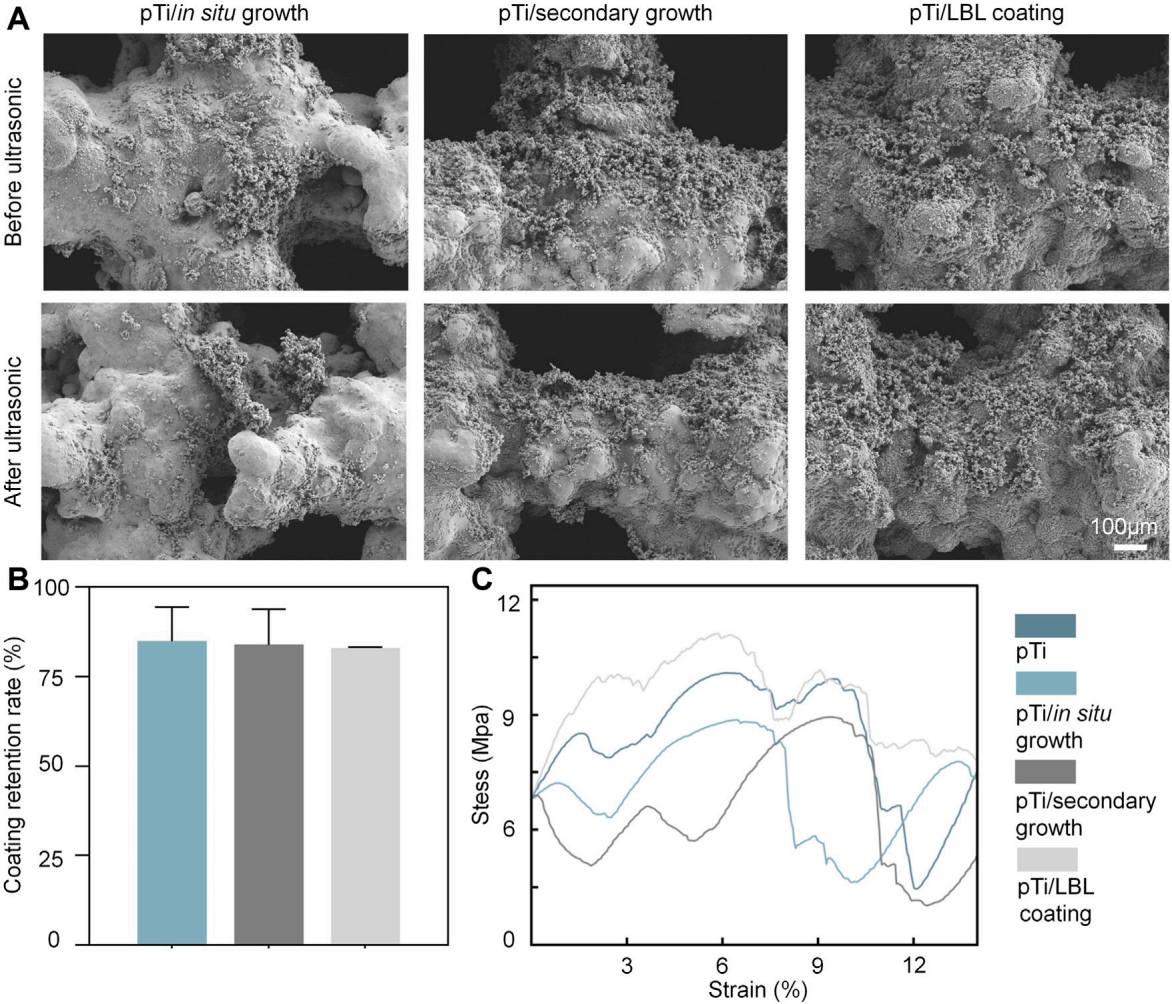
The stability performance of coatings and implants. (A) SEM images of the surface morphology of the pTi-*in situ* growth, pTi-secondary growth, and pTi-LBL coating samples before and after ultrasonic treatment. (B) The coating retention rate of the pTi-*in situ* growth, pTi-secondary growth, and pTi-LBL coating samples. (C) The compressive stress–strain curve of each group (*n* = 3).
